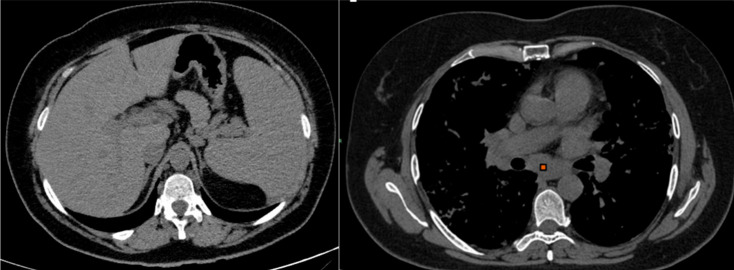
Axial non-contrast CT images show splenomegaly and enlarged subcarinal lymph nodes (red square). The coexistence of splenomegaly, enlarged mediastinal lymph nodes, and interstitial lung disease with a nodular pattern should raise suspicion for an underlying lymphoproliferative disorder.
